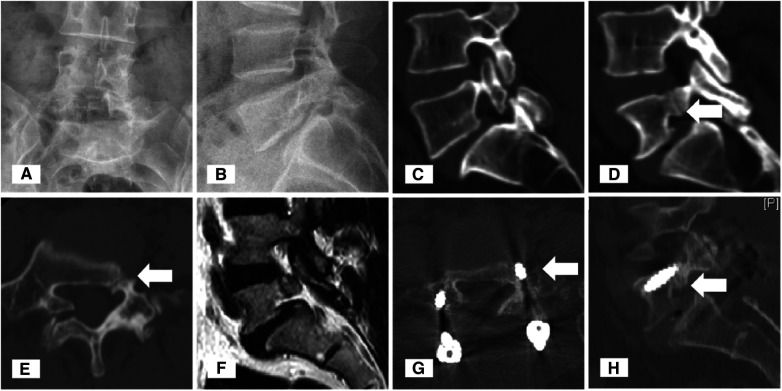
Typical images of Case 2. An anteroposterior plain radiograph revealed the obscure boundary of the L5 left pedicle (A). Lateral plain radiograph revealed Meyerding grade 1 spondylolisthesis (B). Sagittal and axial computed tomography (CT) images of the lumbar spine showed right spondylolysis (C) and right dysplasia (D,E) and the pedicle cleft (white arrowheads) of the L5 vertebra. T2-weighted sagittal magnetic resonance imaging of the left pedicle showed a hypointense signal of the cleft (F). Postoperative CT demonstrated that bony fusion occurred at the preoperative pedicle cleft (G,H) site.
